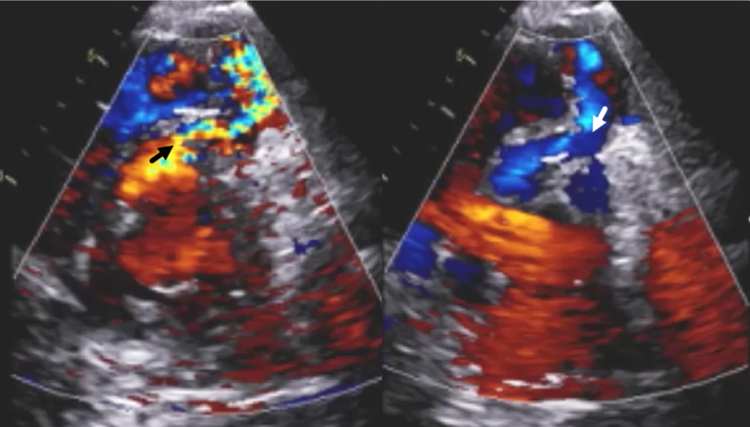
Postintervention TTE Showing To-and-Fro Flow Parasternal mid-LV short-axis views at the level of the midventricle in (Left) systole and (Right) diastole shows to-and-fro flow between the LV and RV dissection cavities. LV = left ventricular; RV = right ventricular; TTE = transthoracic echocardiography.
